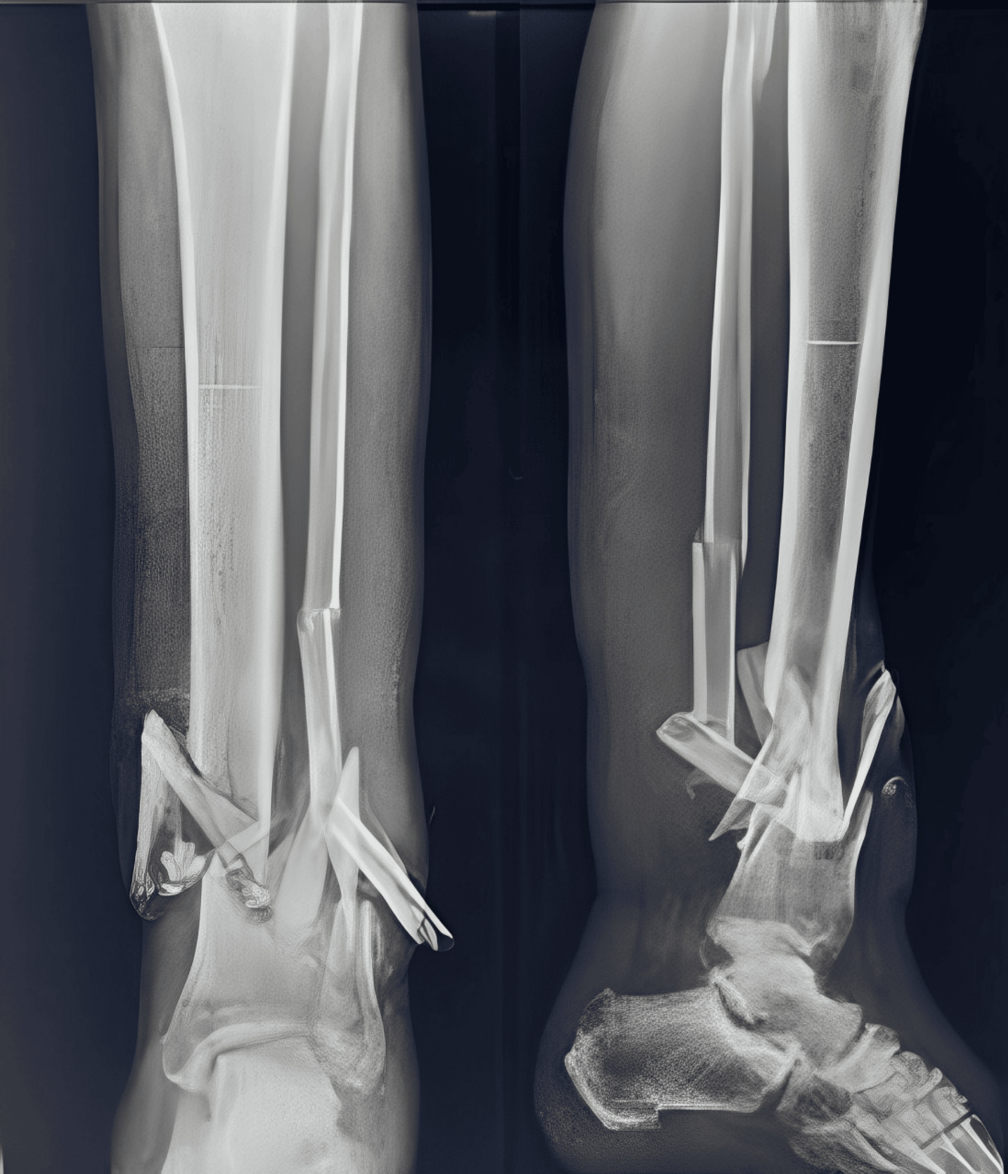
X-ray showing the distal third tibia-fibula fracture with severe communition
